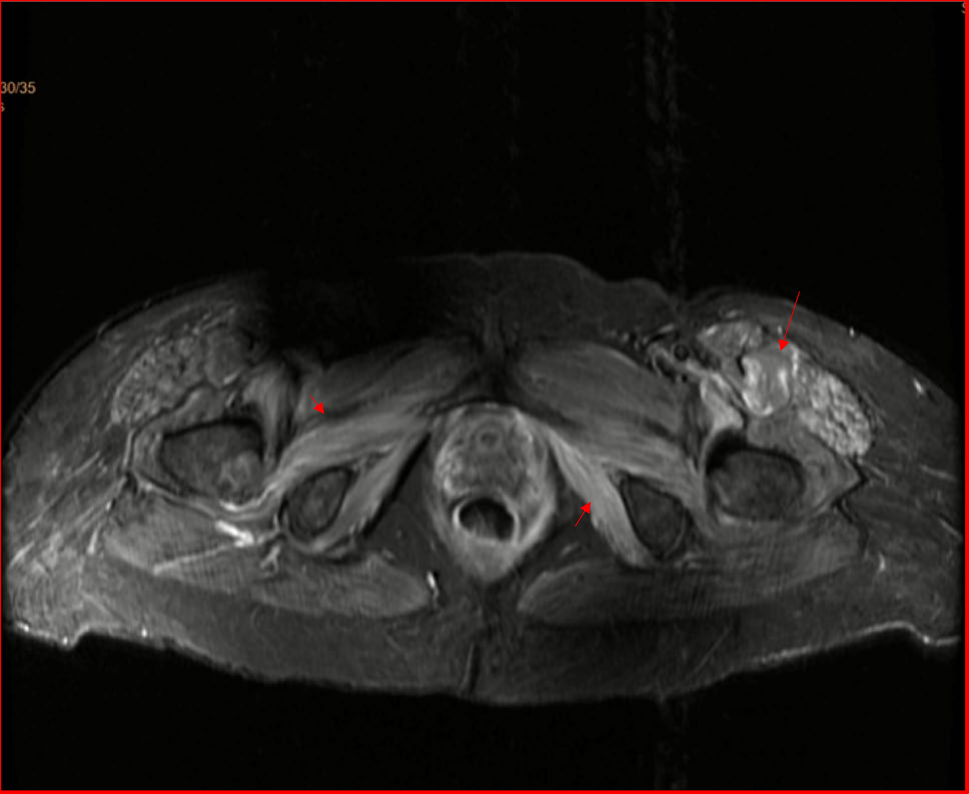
In the same patient, transverse T2-weighted image with fat saturation of the pelvis. There is increased signal of selected muscle compartments, more prominent on the left, indicative of axial skeletal myositis
